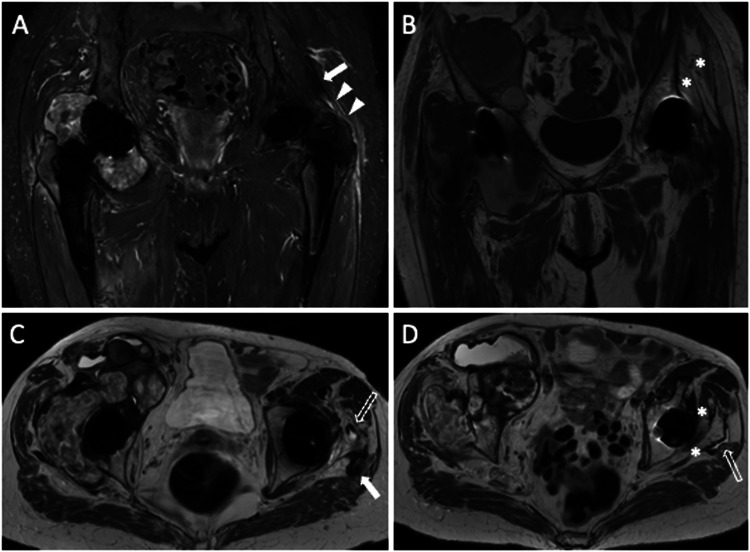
Pelvis MRI of a 69-year-old female patient with bilateral THA and tear of the left gluteus medius tendon. Coronal STIR (A), coronal T1-weighted (B), and axial T2-weighted (C, D) images show the complete tear of the gluteus medius tendon (arrowheads: tendon gap; white arrows: tendon stump), atrophy of gluteus medius and minimus muscles (*). Also, peritendinous edema and subgluteal bursitis are observed (void arrows). Note particle disease in the right THA
